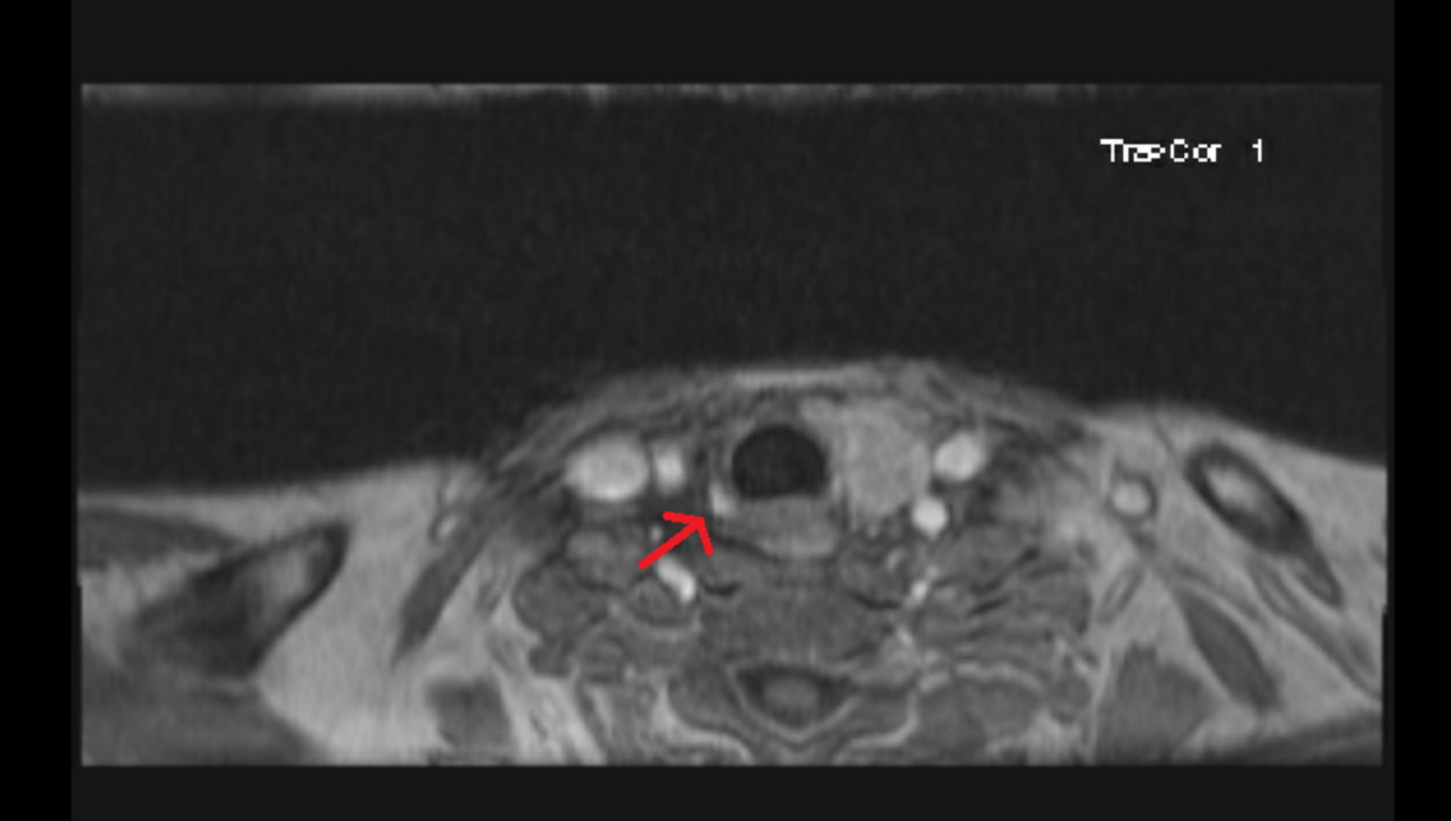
MRI of the neck with contrast showing an arterially enhancing nodule (arrow) in the region of the right thyroid resection bed suggestive of parathyroid adenoma.
